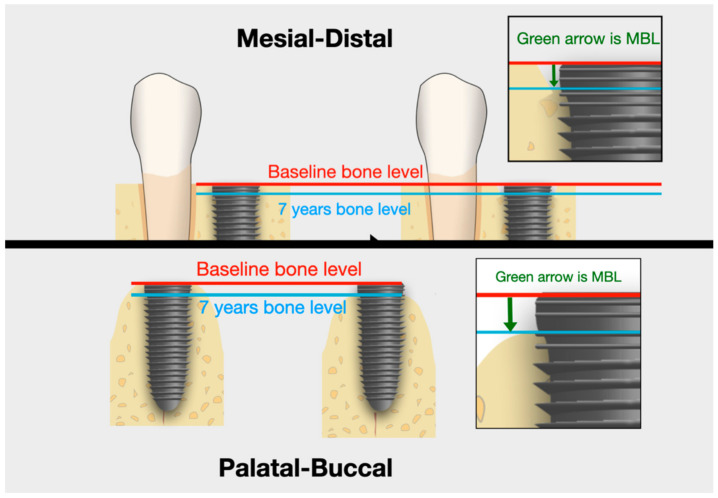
Marginal bone level (MBL) measuring points on CBCT were located around the implant platform (four points around each mesial, distal, buccal, and palatal).
